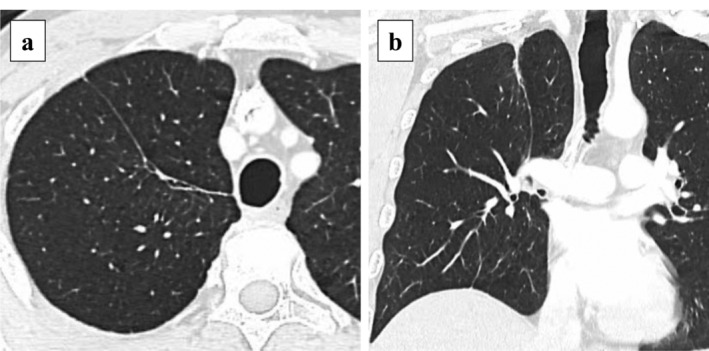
Computed tomography findings at the nine month follow‐up examination after surgical treatment of the secondary pulmonary cyst. (a, b) No new pulmonary cysts had developed.
